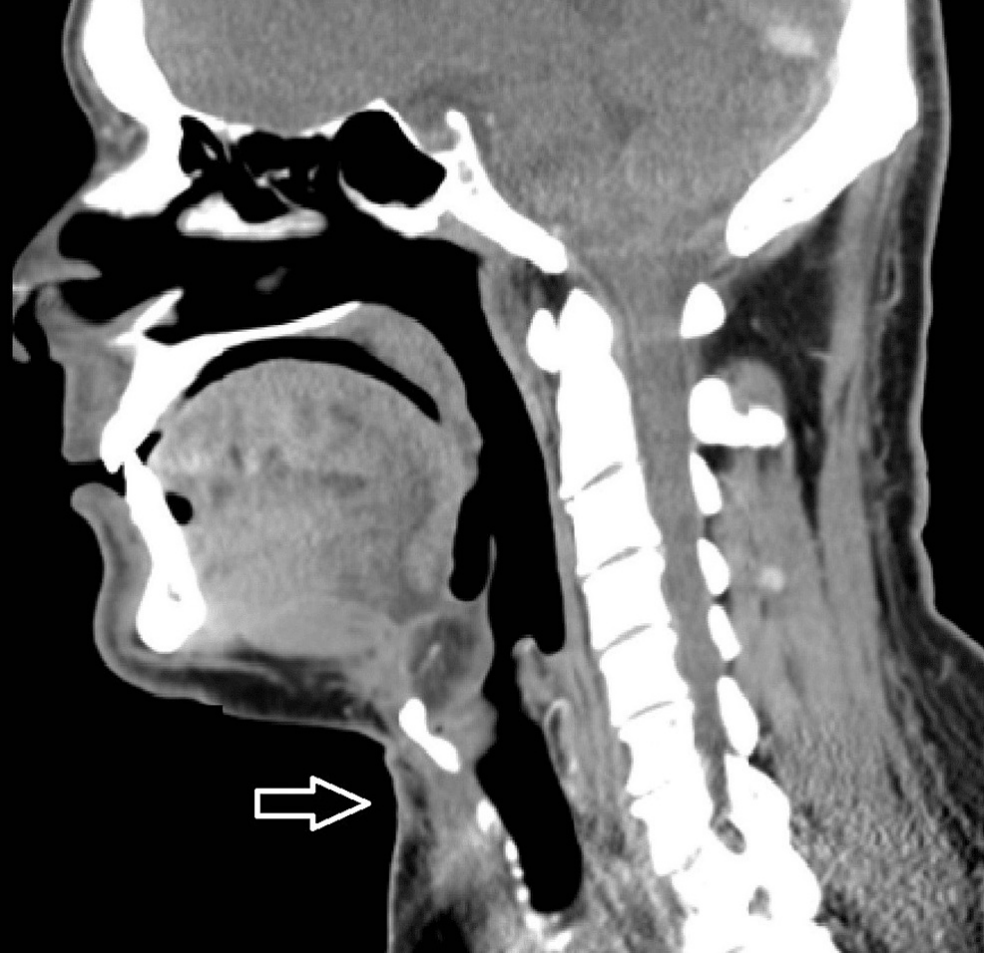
Sagittal CT image of the same patient, one year after surgical treatment, showing removal of the cystic lesion and of the body of the hyoid bone.
